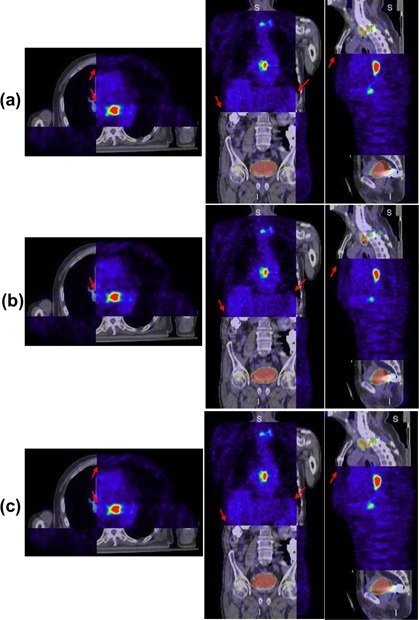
Example of registration results between CT and PET images from Patient #2: (a) PET/CT fused after rigid registration; (b) PET/CT fused after using the GMI‐based demons algorithm; (c) PET/CT fused after the PMI diffeomorphic‐based demons algorithm. The highlighted areas in PET images represented the lesions. Obvious global and local misalignment between PET and CT (indicted by red arrows) could be found before registration. After using the GMI‐based demons algorithm, the misalignment was greatly corrected, except for several small errors (indicted by red arrows). After using the PMI diffeomorphic‐based demons algorithm, PET and CT images registered each other very well.
